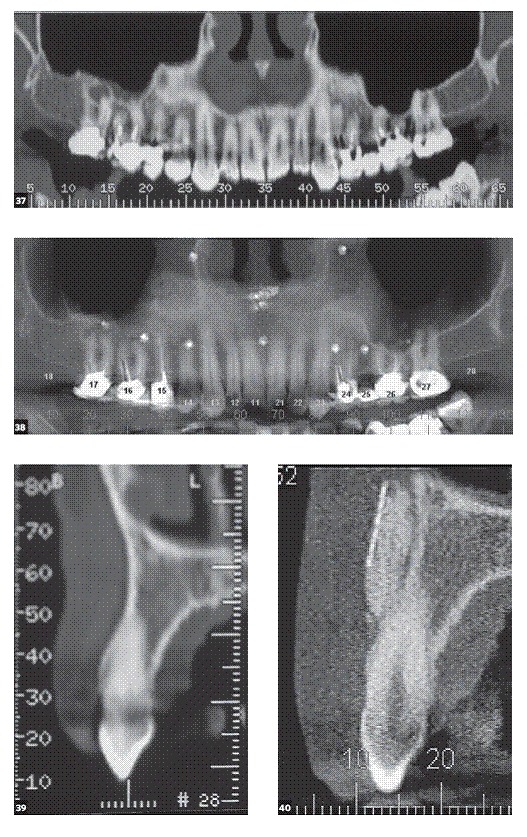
Panoramic view obtained from CT scan taken before surgery. 38) Panoramic view obtained from CT scan after surgery, showing titanium mesh and tacks. 39) Cross sectional image of #13 before surgery. 40) Cross sectional image of #13 after surgery. Note de space above de apex, grafted with Bio-Oss^TM^ under the titanium mesh. 41) 3D reconstruction obtained from CT scan before surgery. 42) 3D reconstruction obtained from CT scan shows accommodation of the titanium mesh and Bio-Oss^TM^ in the grafted area.
